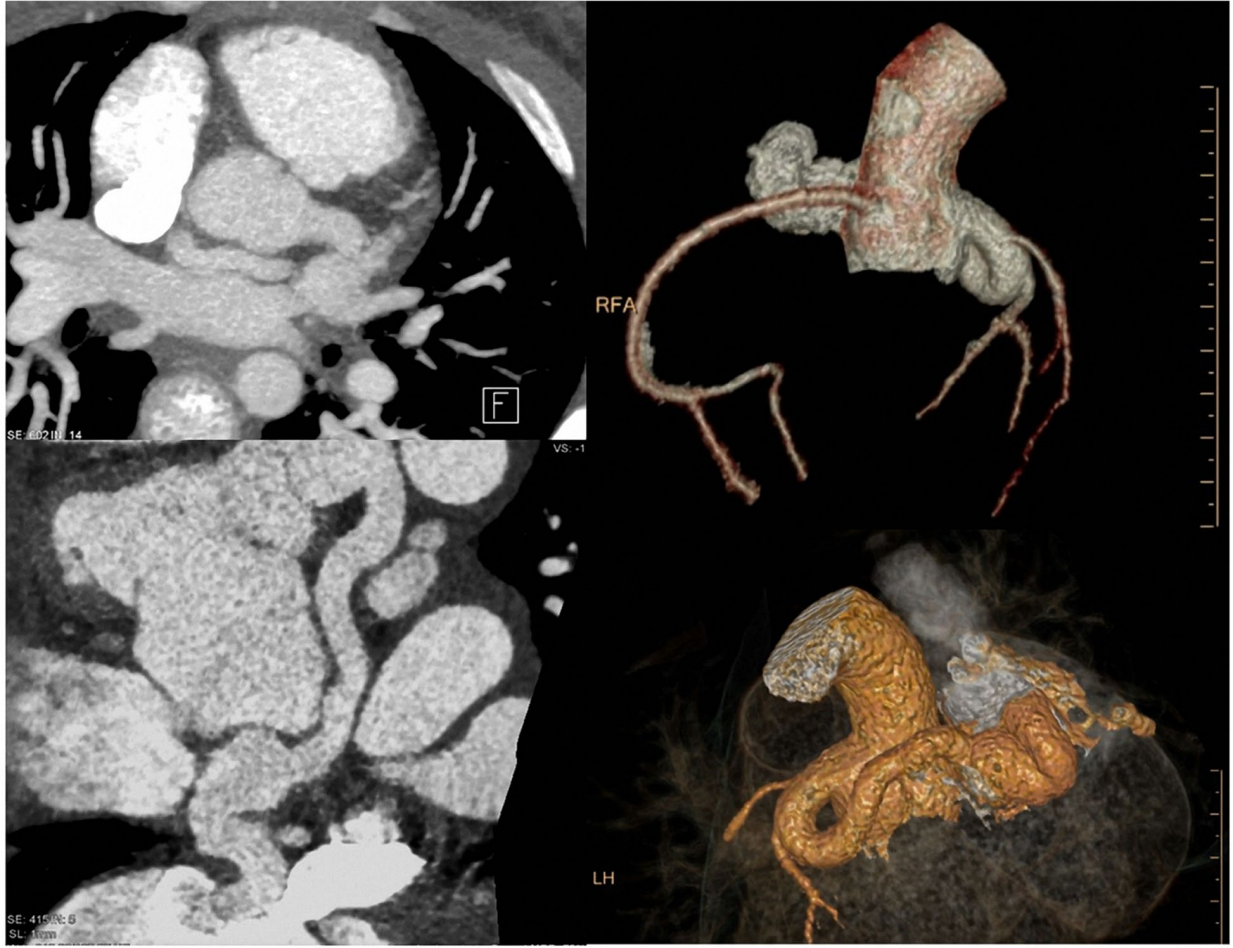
(Planning, dose monitoring (left upper and lower row), axial and paracoronar 2D MIP reconstructions (middle), and 3D VRT reconstructions (right)): 11-year-old girl. Previous surgical repair of a VSD. Now suspected (echocardiography—not shown) coronary fistula. CTCA with dose reduction software, resulting in a low radiation dose (total DLP 63 mGycm ~ 0.8 mSv). CT allows a complete and rapid representation of even highly complex anatomic alterations (in one breathhold)
